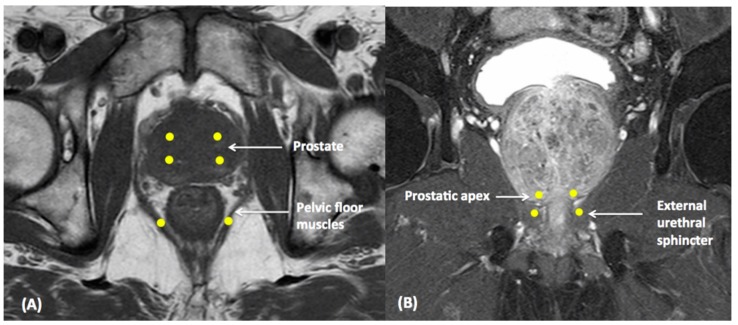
The potential injection sites of BoNT-A for CP/CPPS treatment: intraprostatic, prostatic apex, external urethral sphincter, and pelvic floor muscles. (A) MRI, axial view (B) MRI, coronal view.
